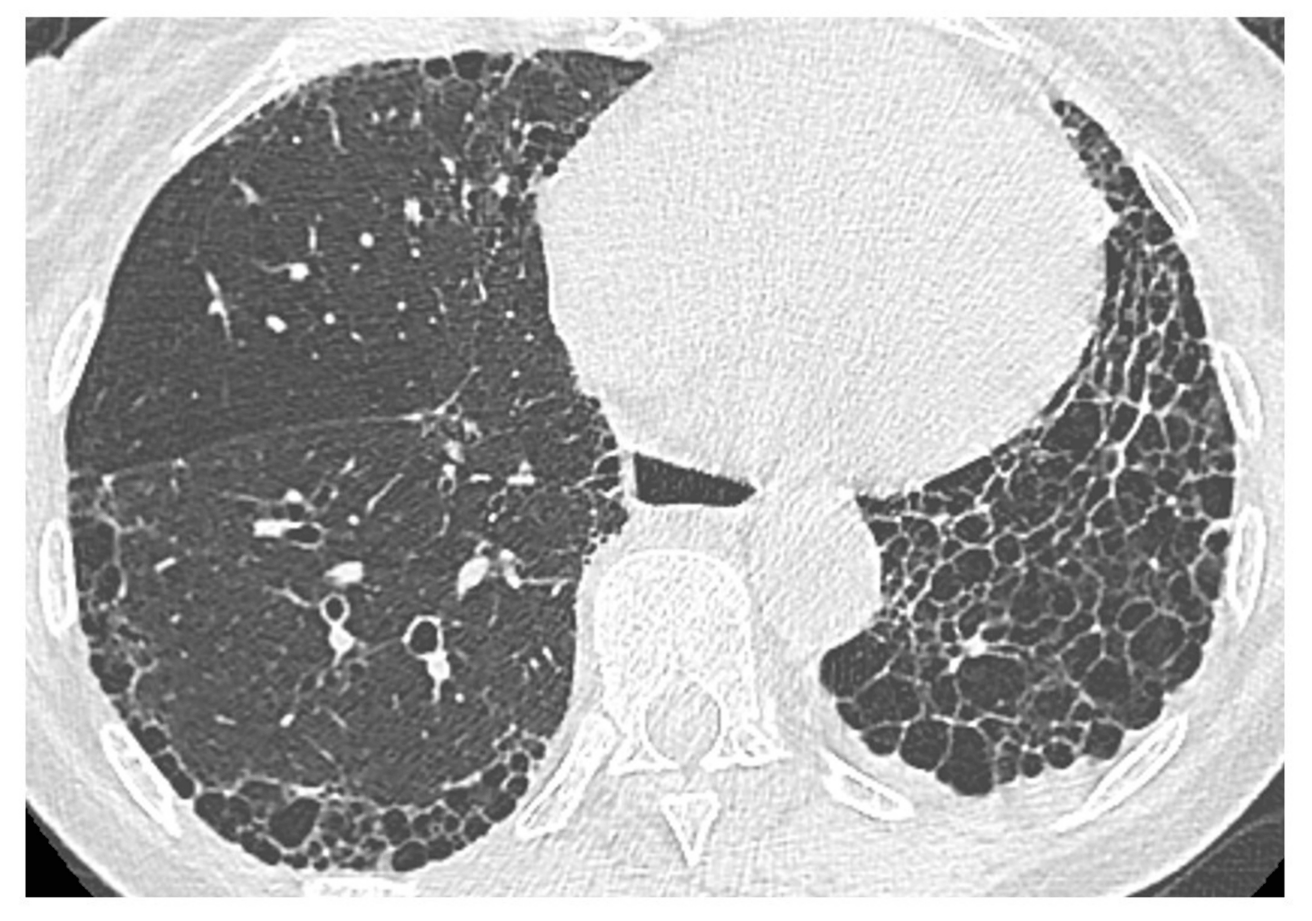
Advanced ILD with pulmonary fibrosis and honeycombing Axial CT image shows a “honeycomb” appearance posteriorly in the right lower lobe and throughout the visualized left lower lobe. Note the small, clustered cystic spaces with well-defined walls. ILD: interstitial lung disease, CT: computed tomography Image credit: Dr. Jordan Ditchek, MD (used with permission)
